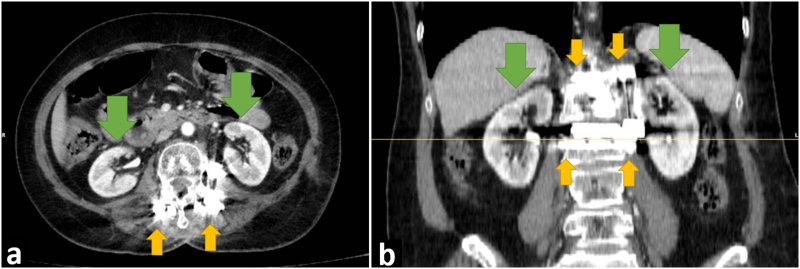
Follow up CT scan 3 months after surgery revealing no tumor progression nor any kidney tumor. Yellow arrows show screw-rod system and green arrows show normal kidneys. a. Transverse section b. Coronal reconstruction of CT scan. Thin yellow line indicates the location of transverse section. (For interpretation of the references to colour in this figure legend, the reader is referred to the web version of this article.)
